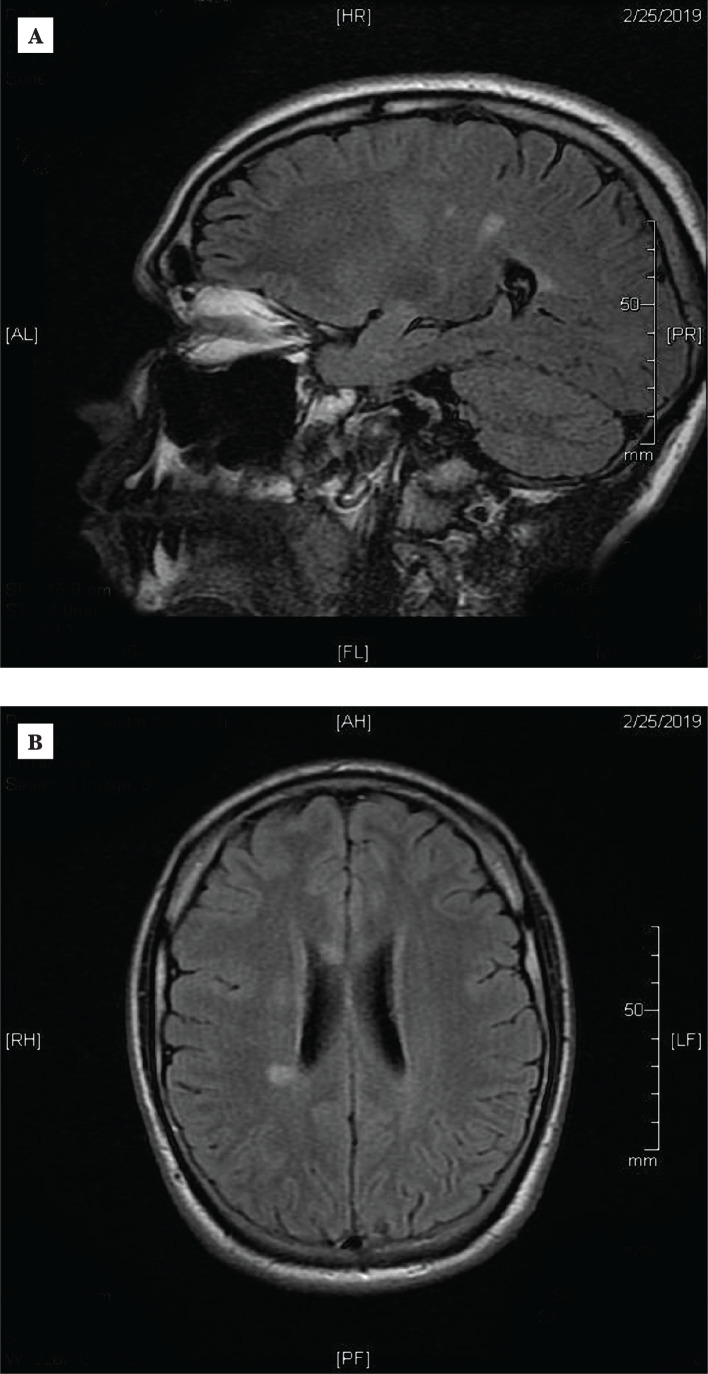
Axial fluid-attenuated inversion recovery (FLAIR) MRI sections revealing hyperintense contrast-enhancing lesions in the corpus callosum and periventricular regions (A and B).
